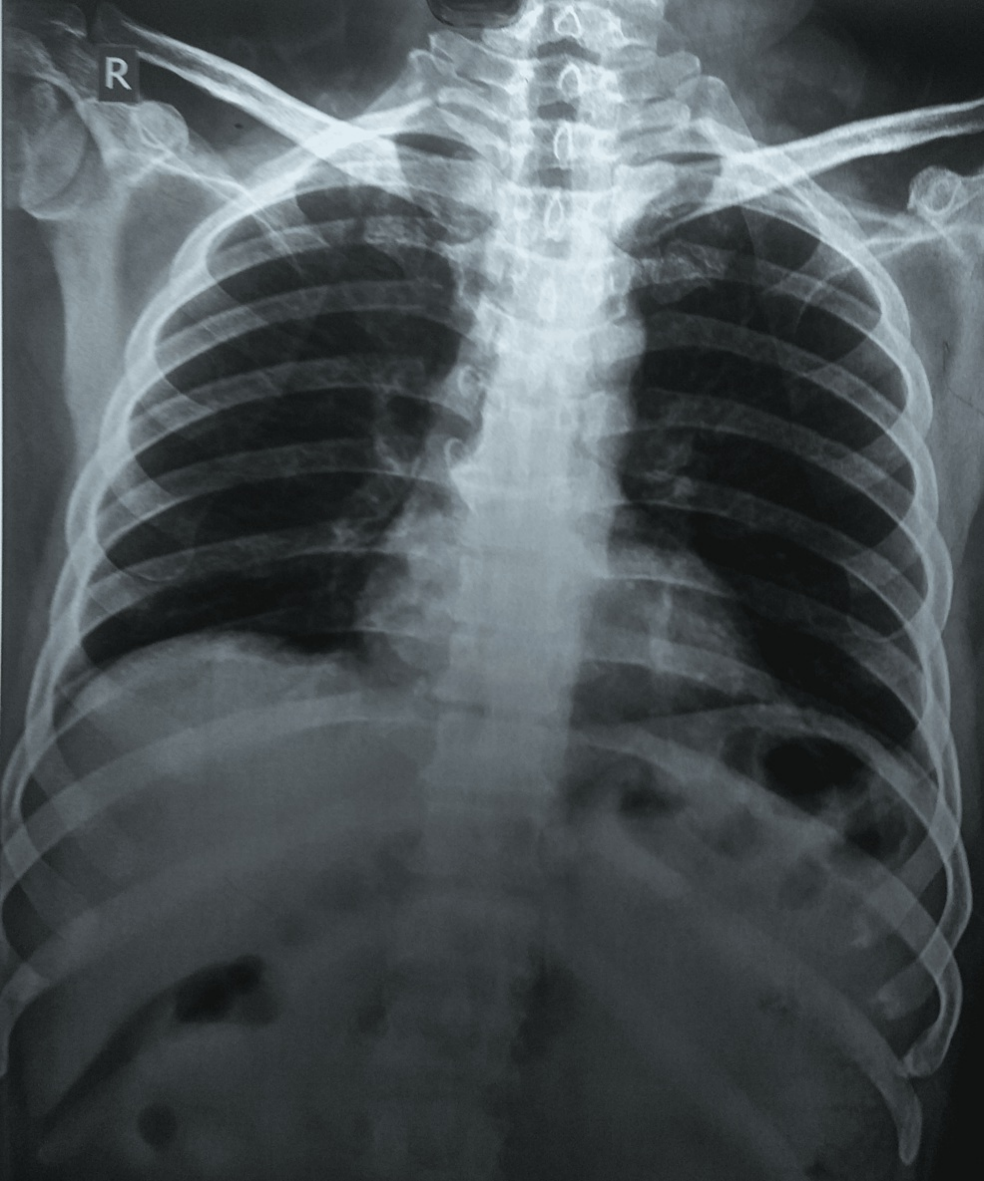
A normal chest radiograph
